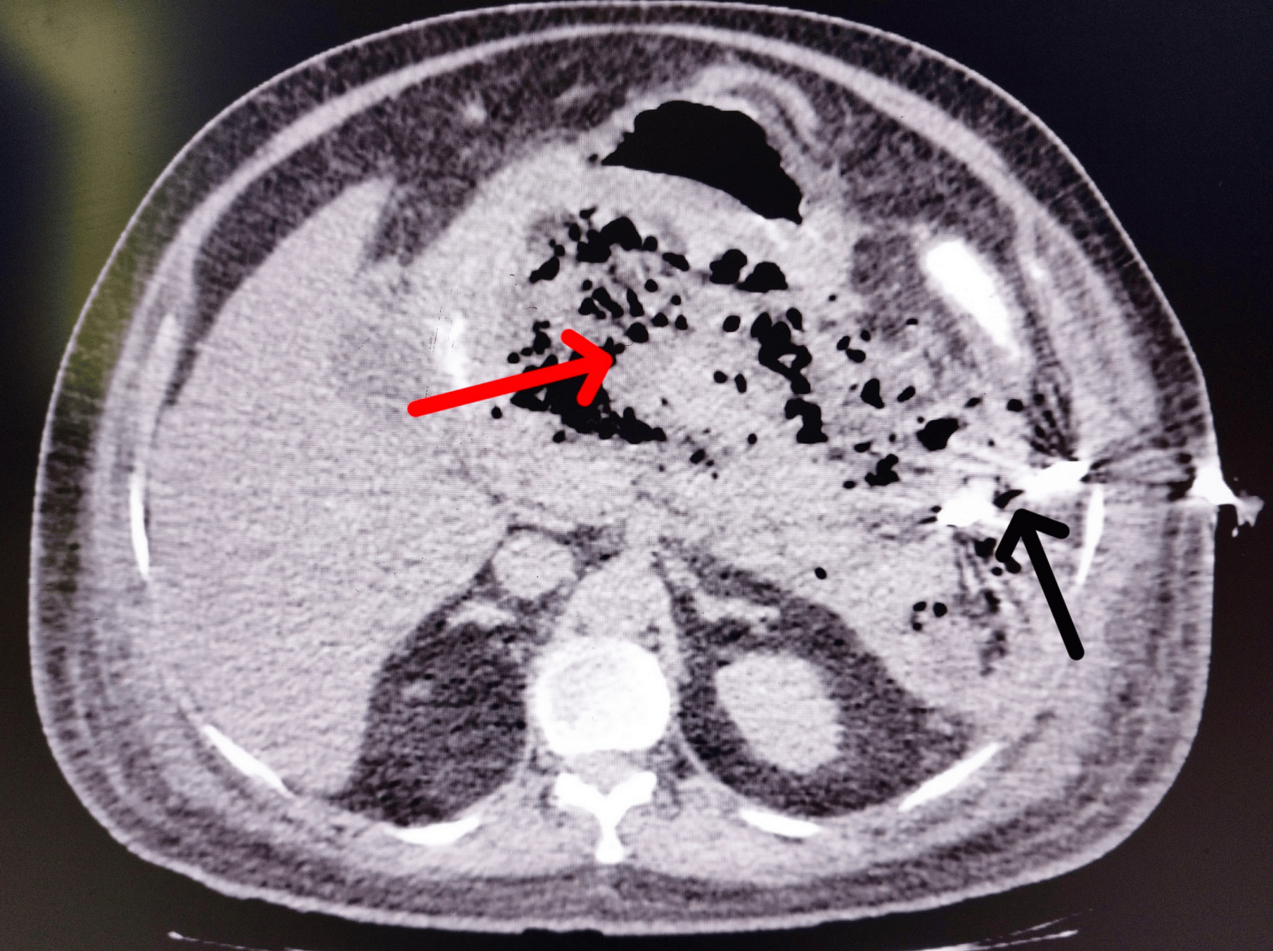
CT scan image showing pancreatic necrosis The red arrow shows a necrotic collection in the region of the head of the pancreas. The black arrow shows a percutaneous drainage catheter draining a necrotic collection in the region of the tail of the pancreas.
